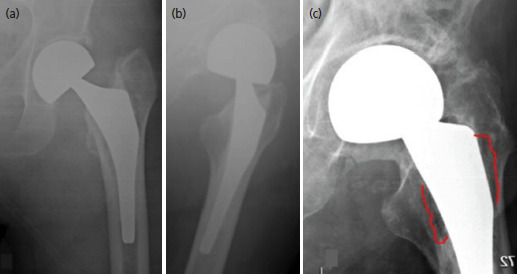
(a) Left hip radiographic anteroposterior view after hemiarthroplasty through anterior approach. (b) Post-operative lateral view. (c) Area of bone resorption (red line) and heterotopic ossification 12 months after surgery.
